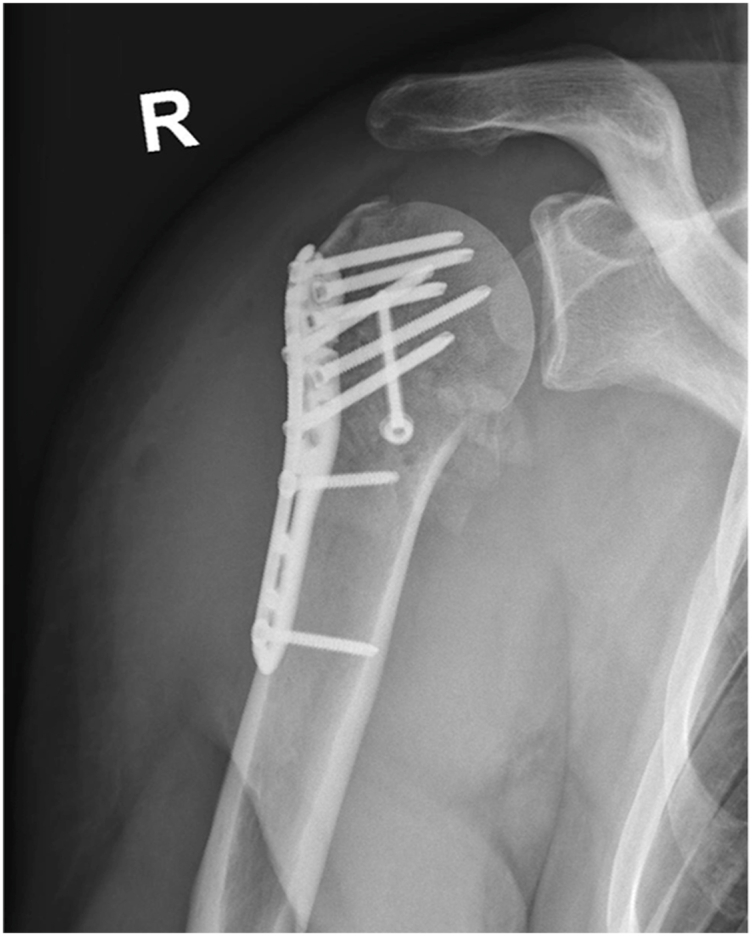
Anteroposterior radiograph of the right shoulder after plate osteosynthesis alio loco.
